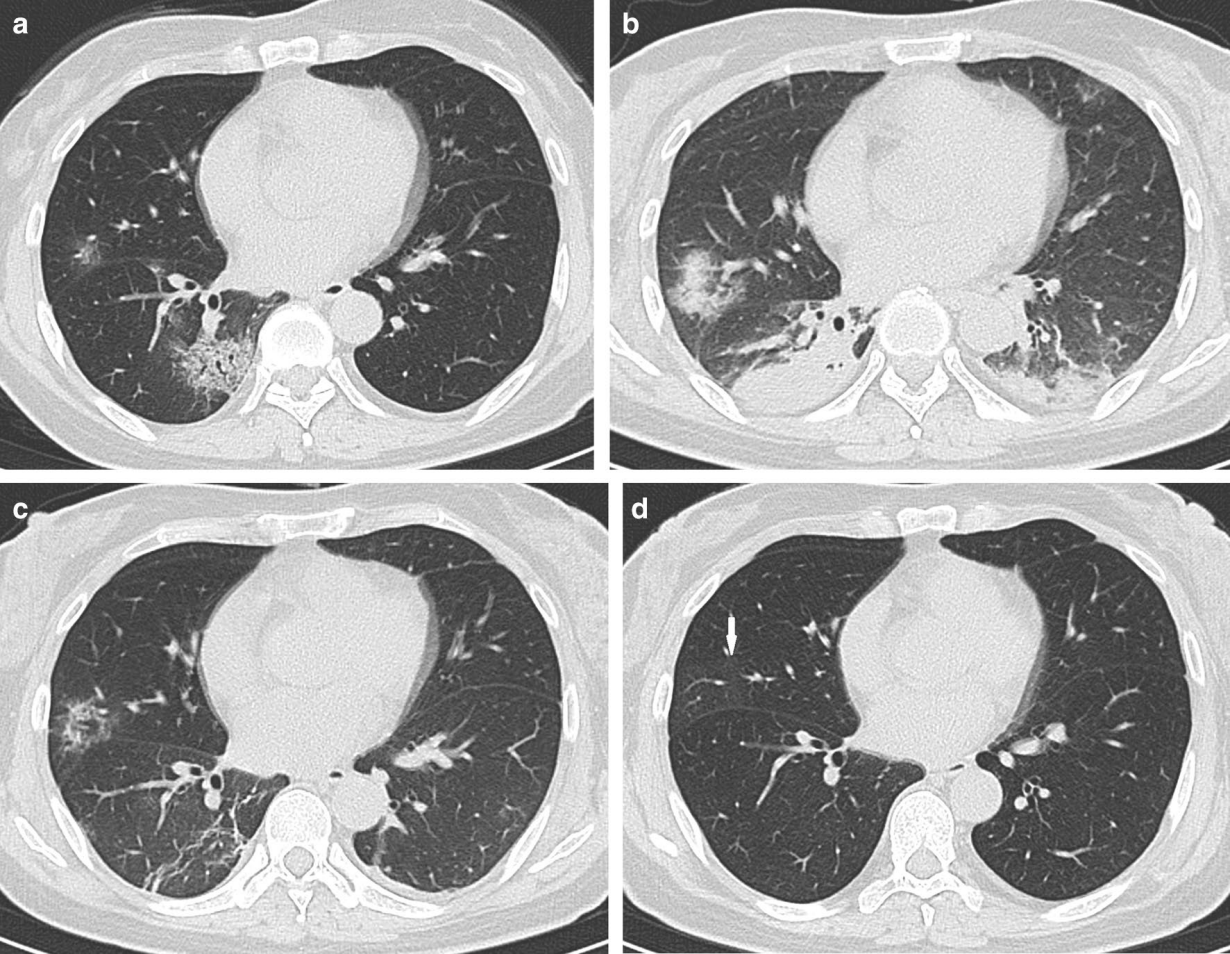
Female, 55-year-old, COVID-19. a The initial CT obtained 5 days from onset of symptoms shows the round ground-glass opacity (arrow) around the subplerual area of the left lower lobe. b The peak CT obtained 9 days later shows the large patchy consolidations and pleural thickening increase much more and air bronchograms are observed. c The pre-discharge CT obtained 8 days later shows consolidation, ground-glass opacity, irregular lines, reticulation, and pleura thickening. d The follow-up CT obtained 45 days later shows a few of ground-glass opacity remained in right middle lobe (arrow)
